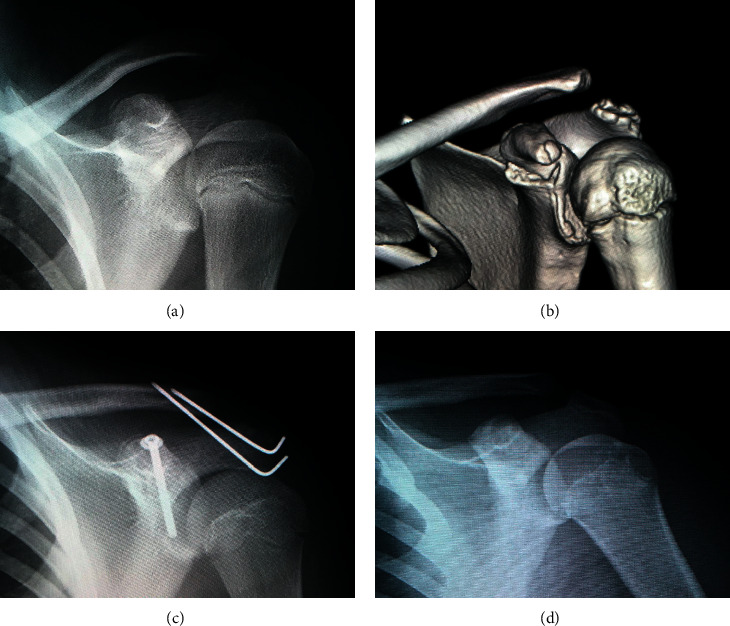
Case 6: representative anteroposterior radiograph: (a) three-dimensional computed tomography reconstruction and (b) Type I injury with an associated acromioclavicular dislocation. Anteroposterior radiograph immediately after the surgery (c) and anteroposterior radiograph three years after the surgery (d).
